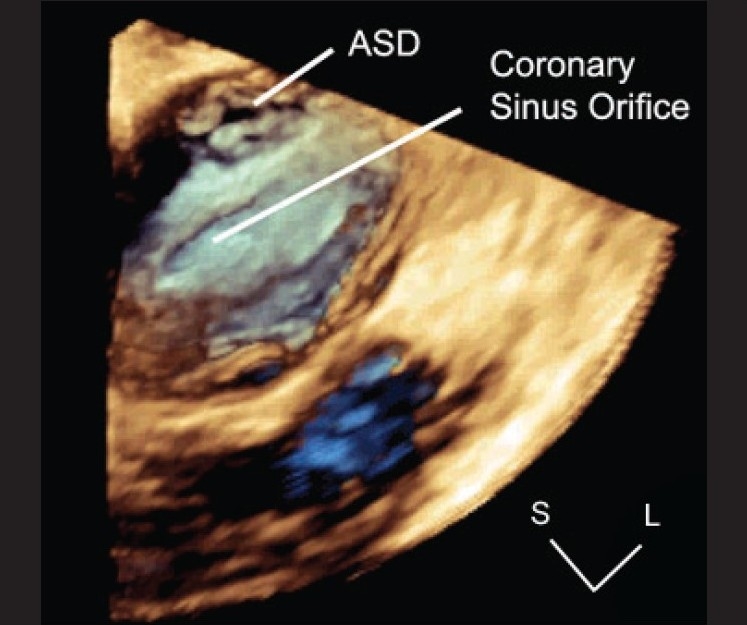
This is a live 3DE transesophageal echocardiogram in a patient with a low secundum atrial septal defect. 2DE imaging demonstrated that the defect was in proximity to the coronary sinus, raising doubts about candidacy for device closure. In this 3DE image, the free walls and appendage of the right atrium have been removed. The viewer is looking posteriorly and leftwards. Note the high resolution of anatomic detail. The atrial septal defect is separated from the coronary sinus orifice by a reasonable distance. Based on the shape and size of the defect, this was felt by 3DE to be a reasonable candidate for device closure, which was successfully accomplished. L, left; S, superior
